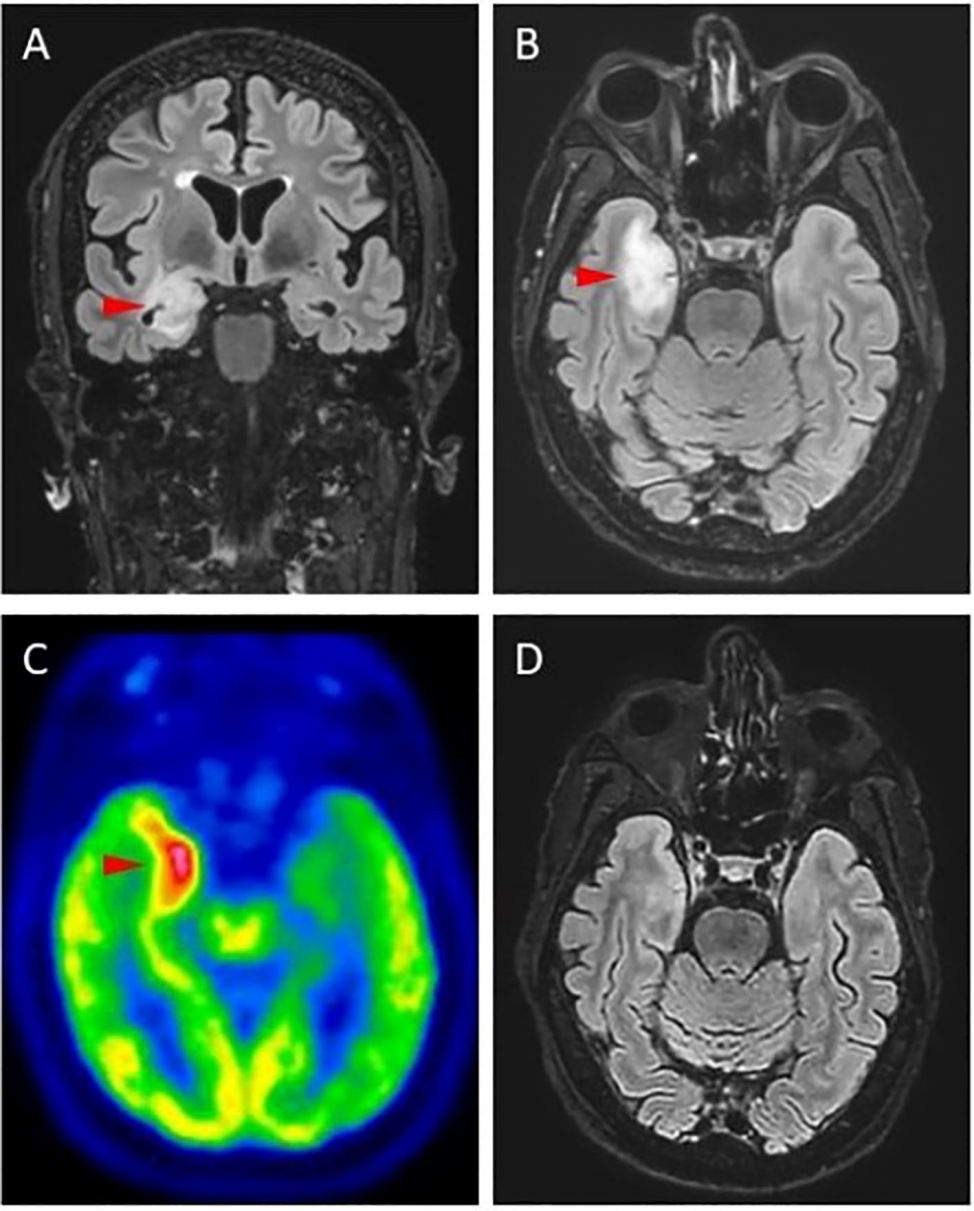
Brain MRI of a patient who developed limbic encephalitis after ICI treatment. (A, B) FLAIR sequences showing hyperintensity and swollen appearance of the right mesial temporal lobe in (A) coronal and (B) axial view. (C) 18-Fluoro-deoxyglugose positron emission tomography (18F-FDG-PET) showing an area of intense tracer uptake corresponding with MRI abnormalities. (D) Control brain MRI performed two weeks after pulse steroids (1 g/die for 5 days) showed complete resolution of the abnormalities.
